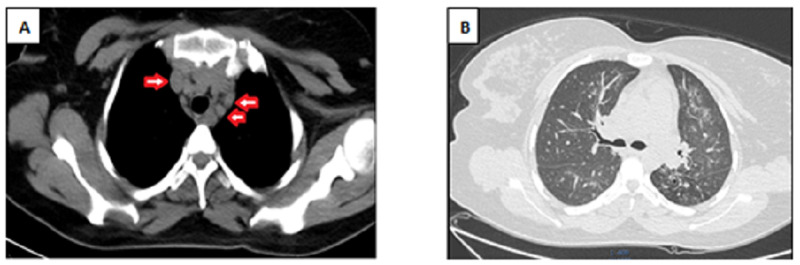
Chest computed tomography showed: (A) Multiple small- and medium-sized discrete non-calcified mediastinal lymphadenopathies (arrow), and (B) Multiple ground glass nodular opacities in both lung fields
